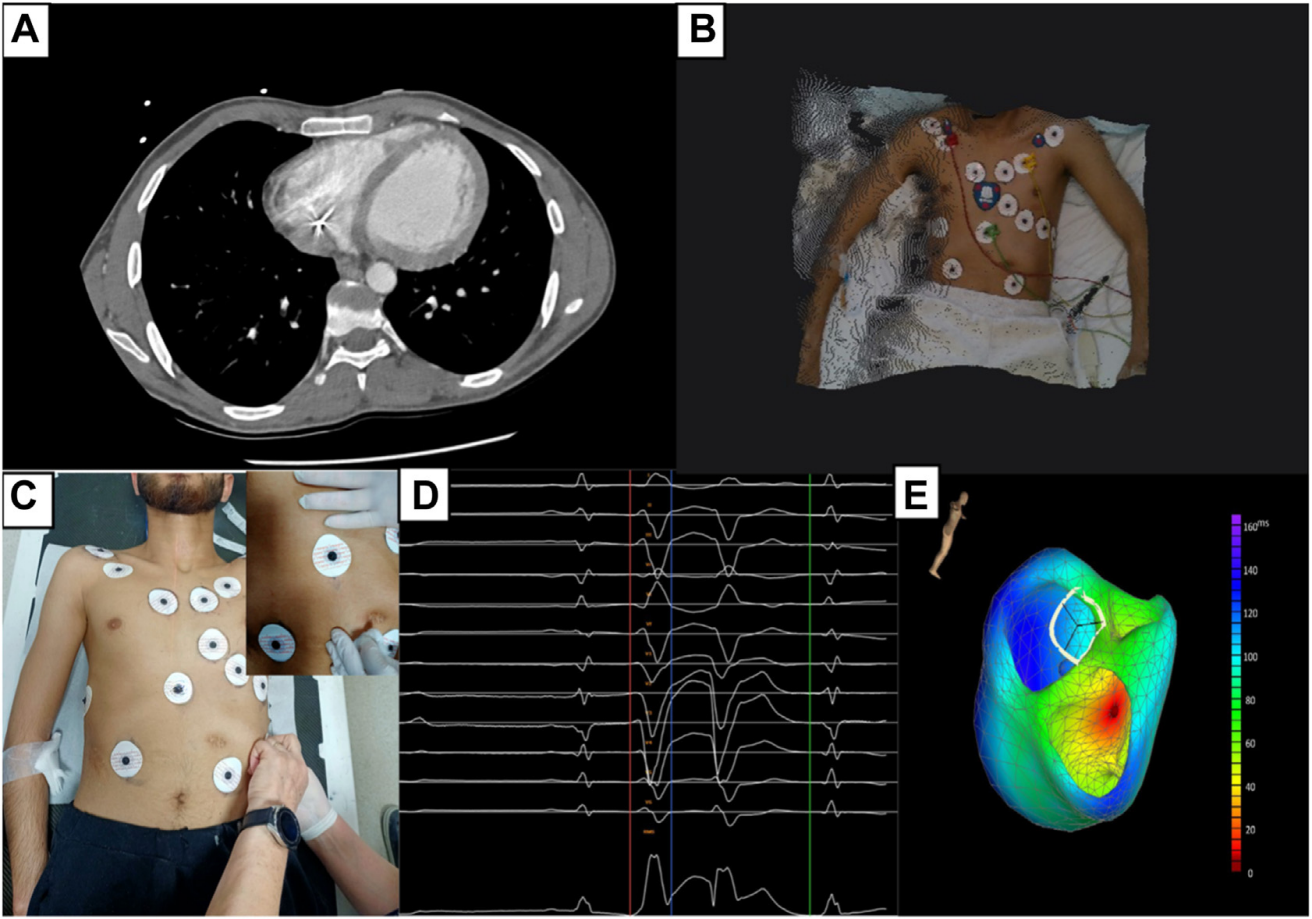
How to collect the premature ventricular contraction (PVC) template in very rare PVC burden with idiopathic ventricular fibrillation for noninvasive activation mapping precision tool. A: Cardiac computed tomography (CT) performed. B: Body 3-dimensional (3D) photograph was obtained via VIVO device with landmark patches. C: Tattoage, which keeps the electrode in the correct position out of hospital, was performed to increase accuracy of 3D body photography. Tattoage was used to mark the electrodes at the 6 o’clock position by needle imprint method. D: PVC collected and transferred to VIVO module.
